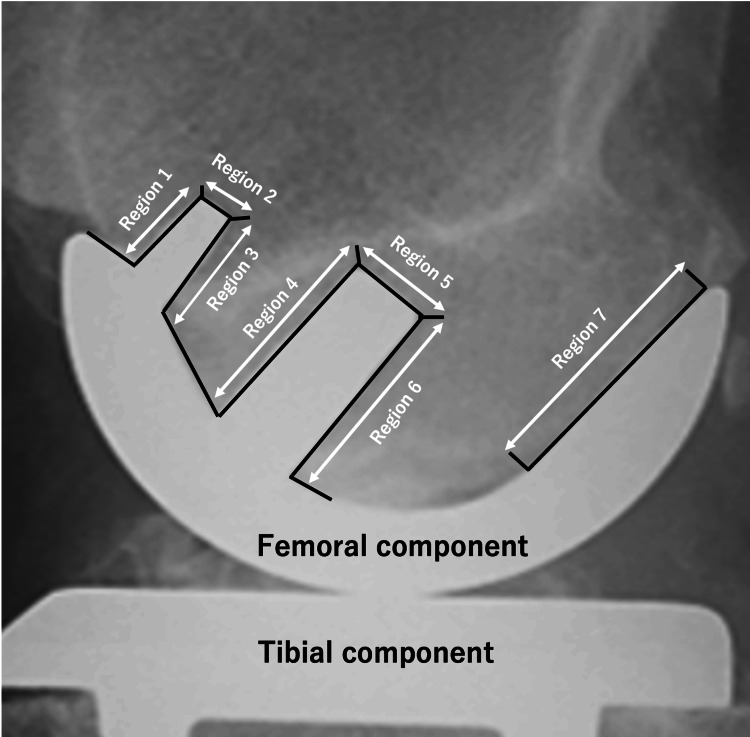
Radiograph sagiital views of femoral component Radiograph showing the seven regions for the distribution of radiolucent lines beneath the femoral component of the Oxford UKA UKA - unicompartmental knee arthroplasty
